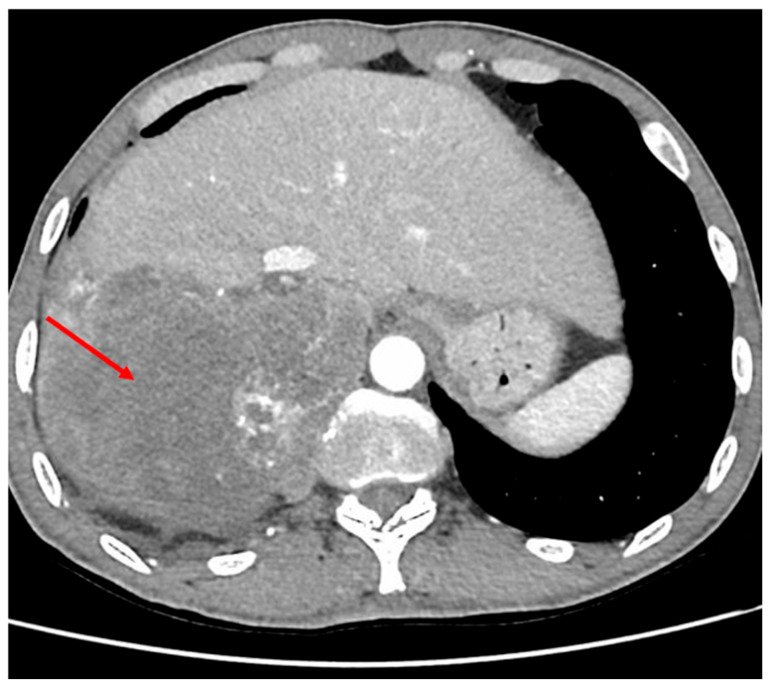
Coronal contrast-enhanced abdominal CT scan demonstrating the adrenal mass displacing the liver and inferior vena cava. No evidence of pulmonary, mediastinal, pleural, or bone metastases. Hormonal workup was conducted to exclude functional adrenal tumors [1]. Serum cortisol, 24 h urinary free cortisol, and ACTH levels were normal, ruling out Cushing’s syndrome. Plasma and urinary metanephrines were within normal limits, excluding pheochromocytoma. The aldosterone-to-renin ratio was also normal, eliminating hyperaldosteronism. Serum dehydroepiandrosterone sulfate, androstenedione, testosterone, and 17-hydroxyprogesterone levels were all within normal reference ranges, further supporting the non-functional status of the tumor.
